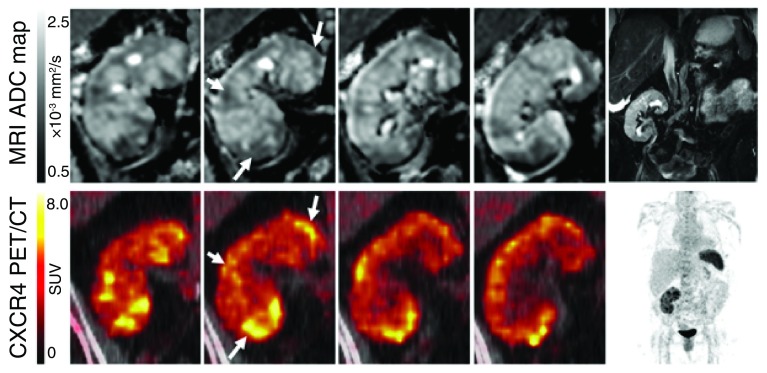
Magnetic resonance imaging (MRI) and CXCR4-positron emission tomography (PET) imaging of acute renal allograft infection. Diffusion-weighted MRI and PET imaging with a specific CXCR4 ligand (
